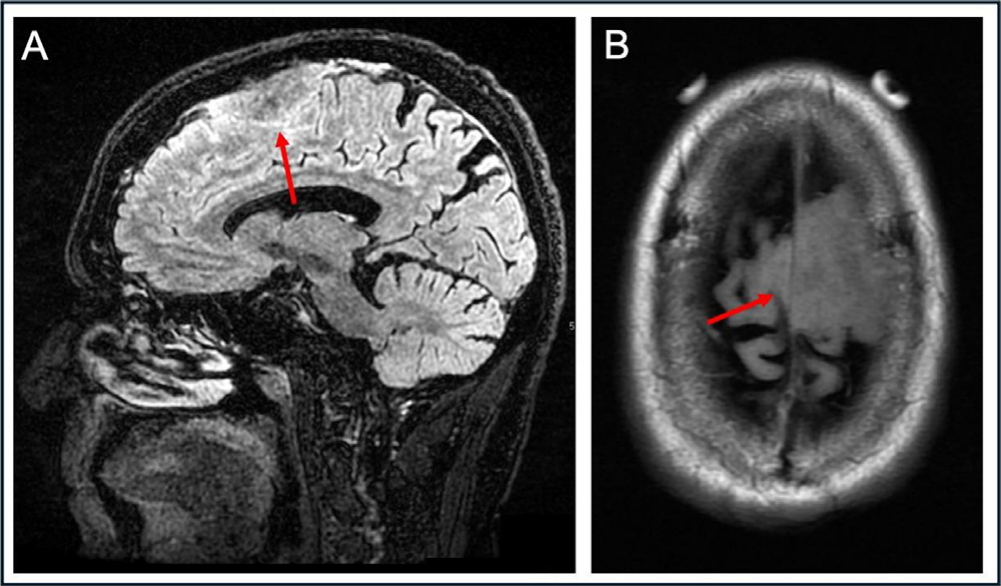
Preoperative sagittal T2 FLAIR (A) and axial (B) 3D T1 MRI of the brain. Overlying the left frontal convexity, there is an extra-axial mass which is mildly T2 hyperintense, crosses midline to the right hemispheric convexity, and invades the adjacent superior sagittal sinus (red arrows). The lesion measured approximately 5.4 × 4.2 × 2.0 cm (transverse, anterior-posterior, and cranial-caudal dimensions). The patient was planned for bifrontal craniotomy for removal of the dural-based lesion 2 weeks after identification on MRI. Pathology revealed a WHO grade 1 meningioma with invasion of the superior sagittal sinus. The patient did not receive intravenous contrast preoperatively due to concerns of impaired renal function.
